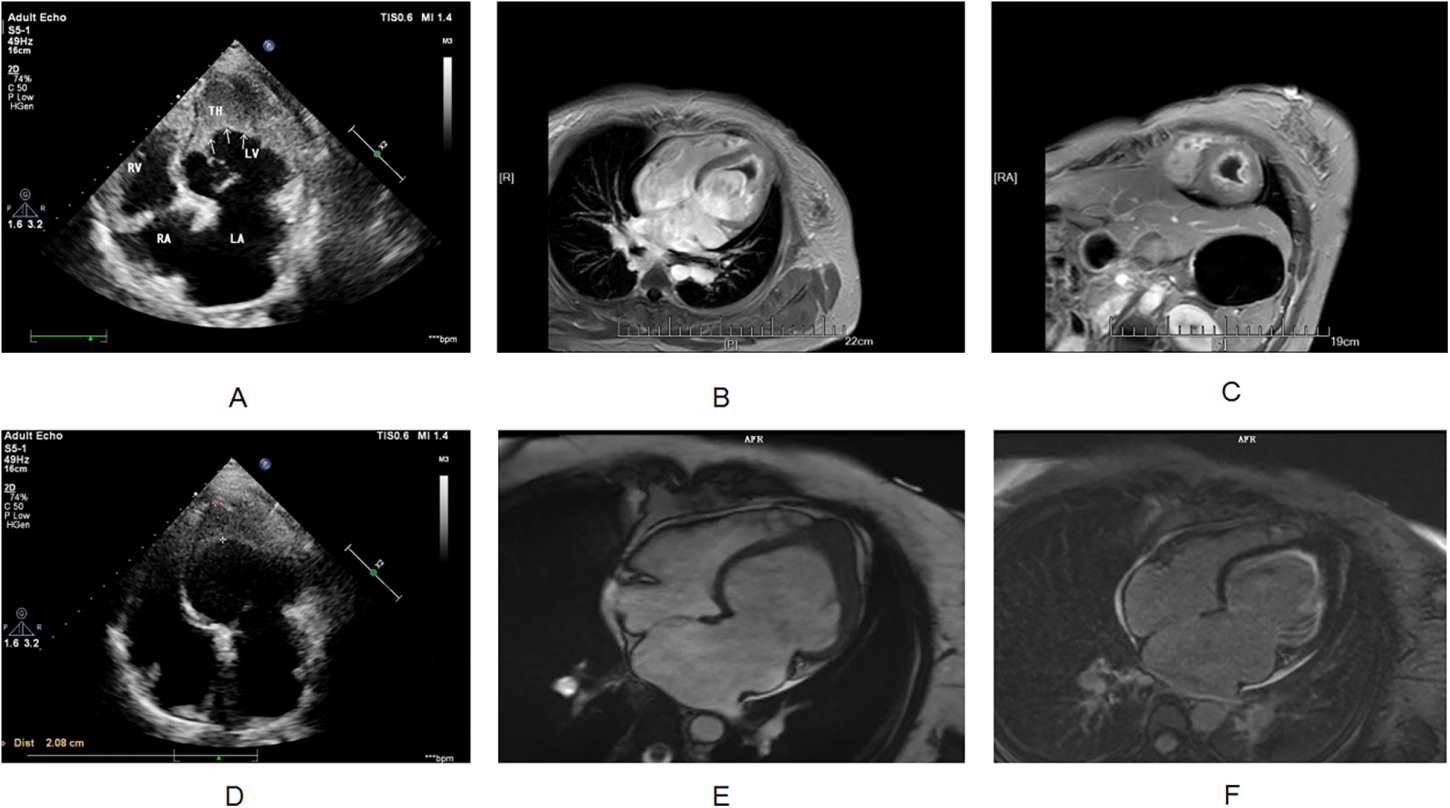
(A) Transthoracic echocardiography shows apical occlusion and numerous mid to high echogenic masses in the lower left ventricular cavity. (B,C) Cardiac magnetic resonance imaging shows occlusion of the apical segment of the left ventricle, and myocardial delayed enhancement imaging shows diffuse enhancement lesions beneath the endocardium of the left ventricle. The signal of the cardiac mass is not enhanced. (D) After hormone combined with warfarin treatment, apical occlusion was still observed on transthoracic echocardiography, and the number of hyperechoic masses in the lower left ventricular cavity decreased compared to before. (E,F) After one year of treatment with imatinib, cardiac magnetic resonance imaging showed apical occlusion, myocardial delayed enhancement imaging showed subendocardial enhancement in the left ventricle, and the cardiac mass completely disappeared.
